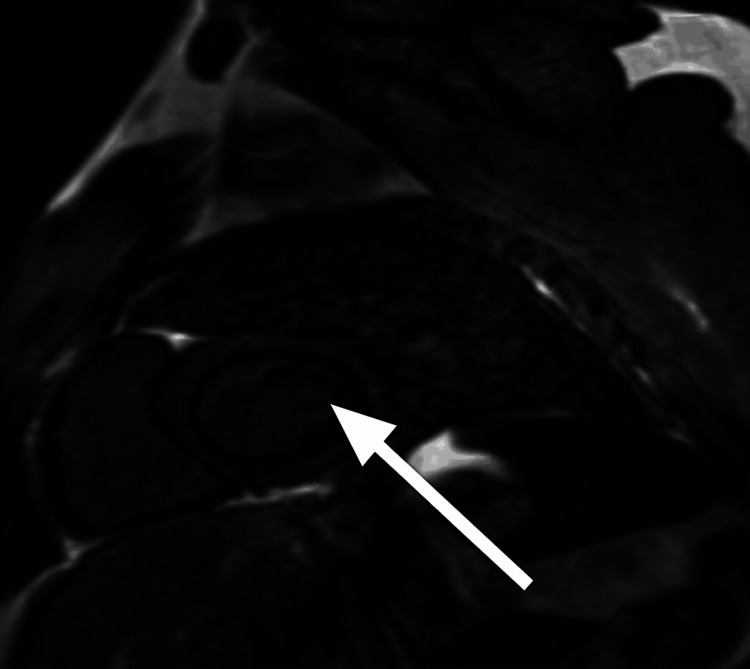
Cardiac magnetic resonance imaging (MRI) Short-axis view showing the left ventricle with myocardial involvement (arrow).
